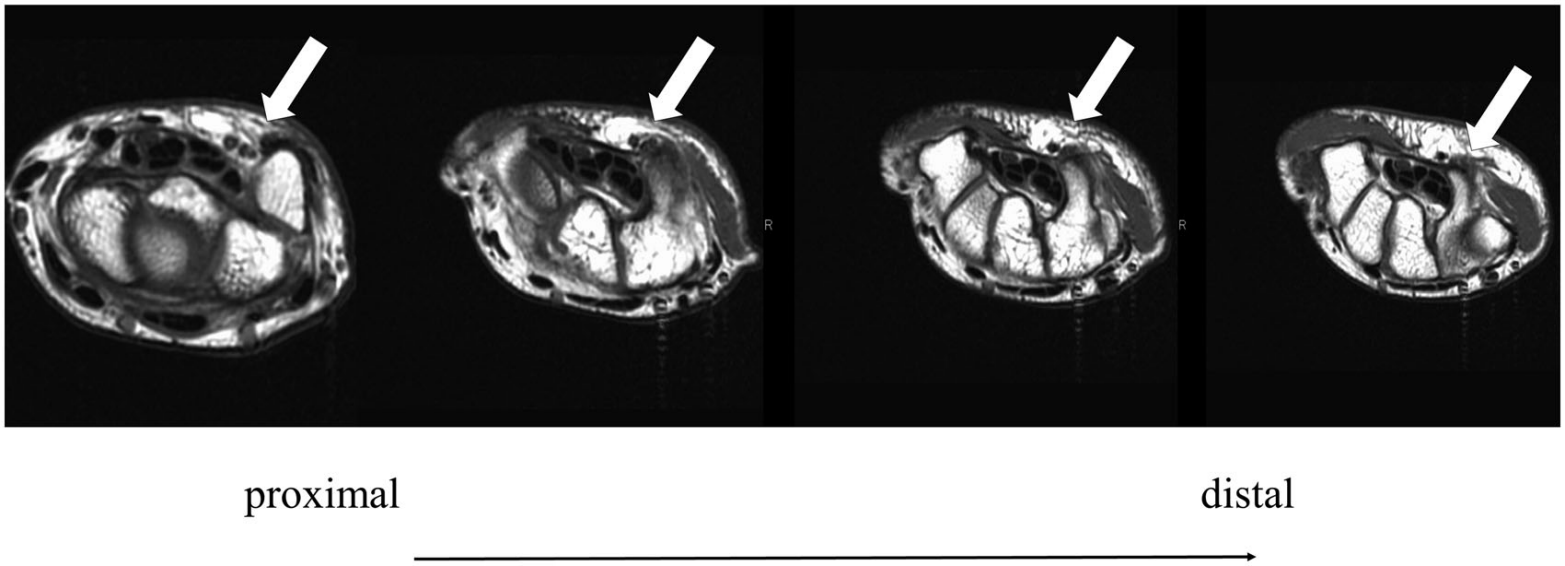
MRI findings revealed that the ulnar nerve (white arrow) run over the hook of hamate.
